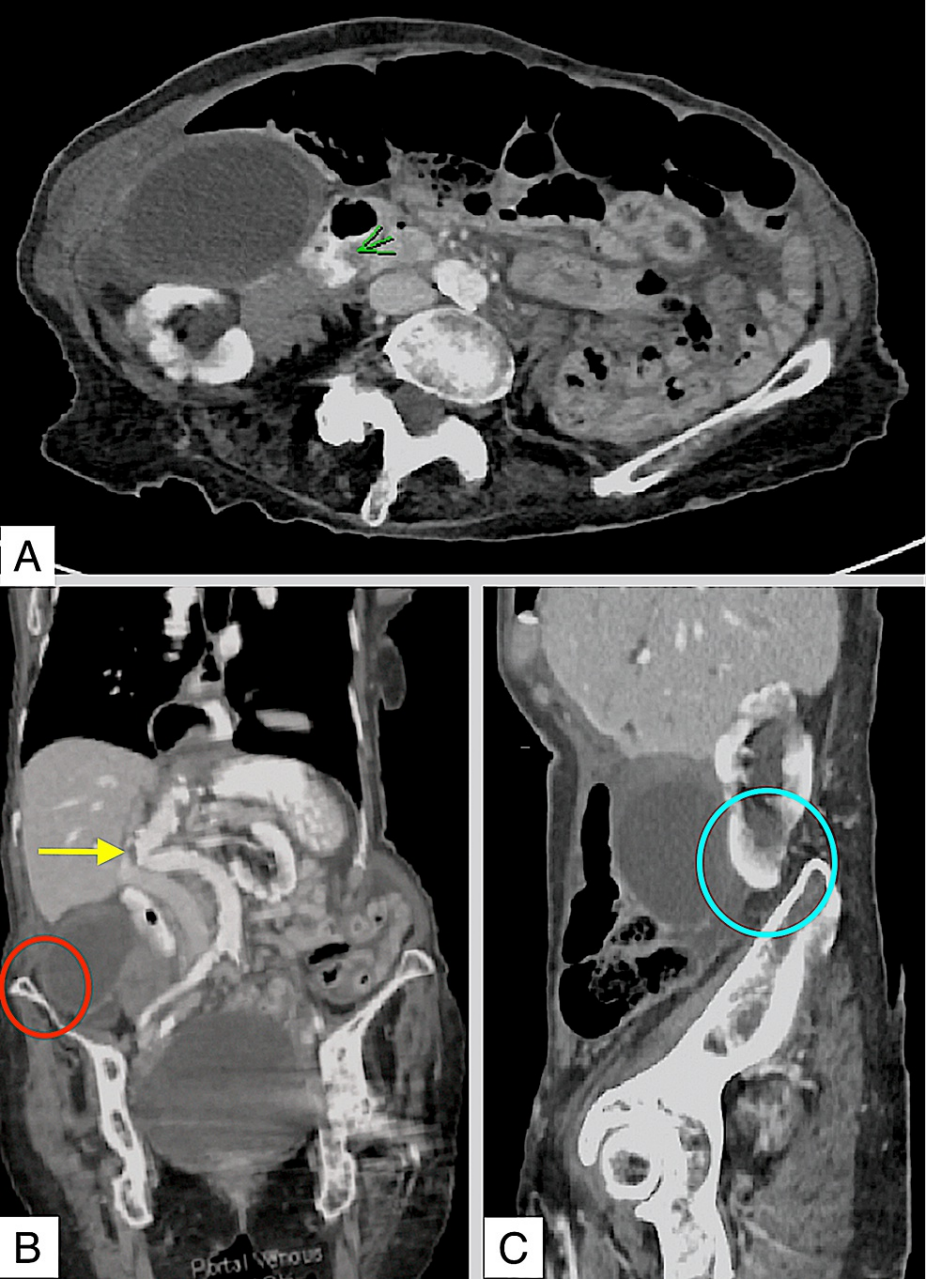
Computed tomography of the abdomen and pelvis with oral and intravenous contrast. (A) Axial computed tomography image demonstrating an ill-defined heterogeneous density within the cystic duct likely an obstructing focus (green arrow). (B) Coronal computed tomography image demonstrating the degree of scoliosis and aortic kinking (yellow arrow) as well as the proximity of the gallbladder fundus to the ilium (red circle). (C) Non-weight bearing sagittal computed tomography image shows the right kidney lying close to the right posterior iliac crest (blue circle).
